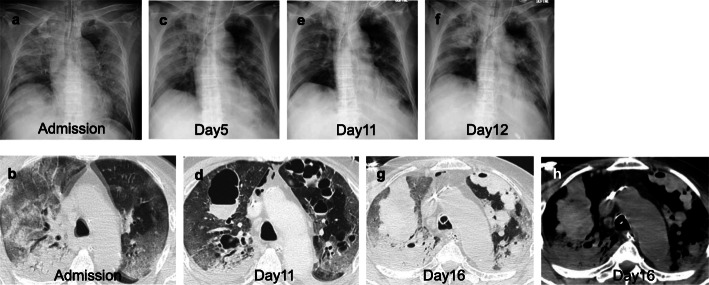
a Chest radiograph demonstrates diffuse ground glass opacities (GGOs) and consolidation in the bilateral lung fields. b CT shows widespread GGOs and consolidation in the dorsal lungs. c, e Follow-up chest radiographs reveal improvement in GGOs and consolidation in the upper and middle lung fields. Multiple thin-walled cysts appear in both middle lung fields (e). d On CT, GGOs and consolidation are improved, and multiple thin-walled cysts containing a small amount of fluid are seen in both upper lobes. f Bilateral cysts have turned into a mass-like opacity. g, h CT shows enlargement of the cysts and exacerbation of GGOs and consolidation in the background of the lungs. Fluid in the cysts show high attenuation, suggesting bleeding
